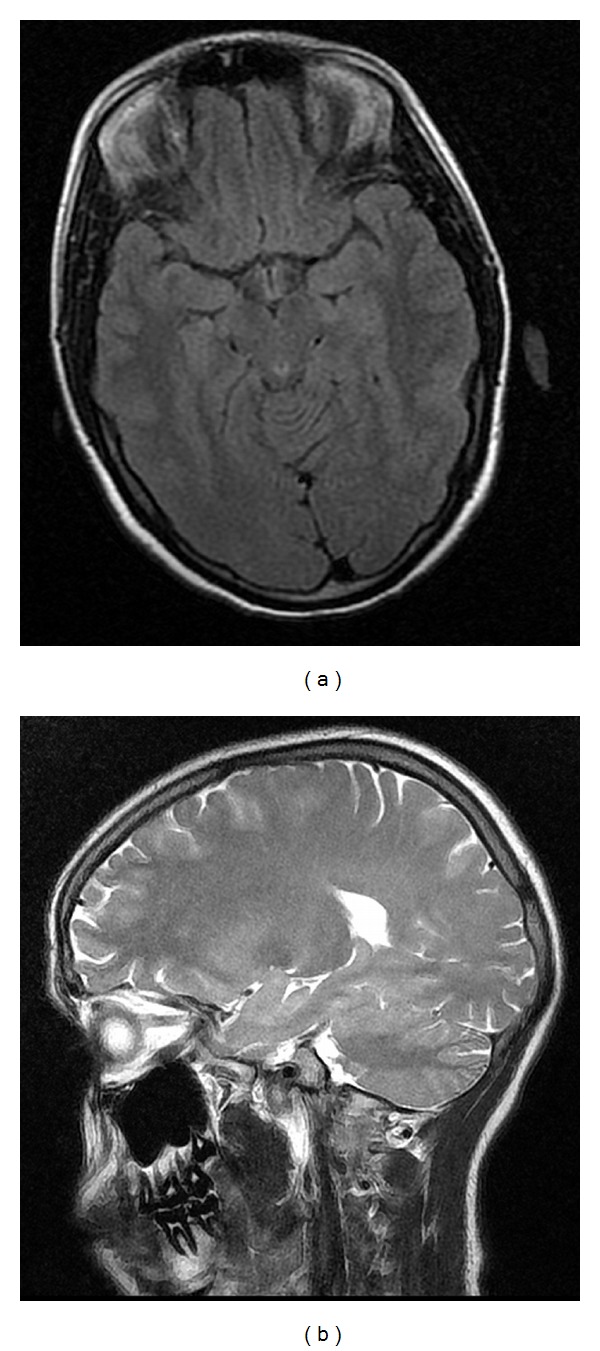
The patient MRI findings in one month later.
